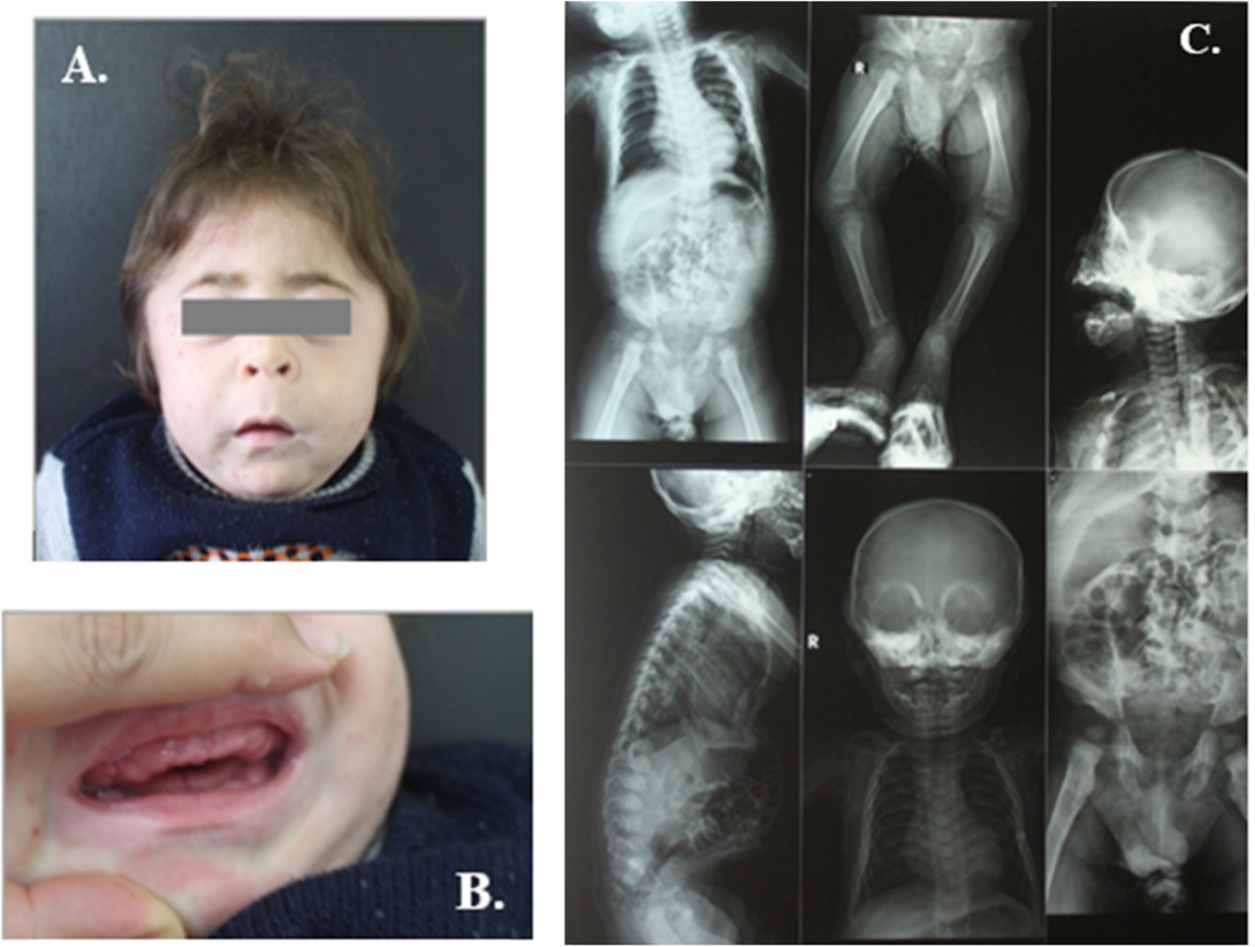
Patient no. 1 at the age of 9 months. A Clinical features of ML II including coarse facial features, long philtrum, tower skull, and short neck. B Hypertrophic gingiva of the mouth. C X-rays of patient no. 1 showing dysostosis multiplex at ends of long bones and vertebrae
